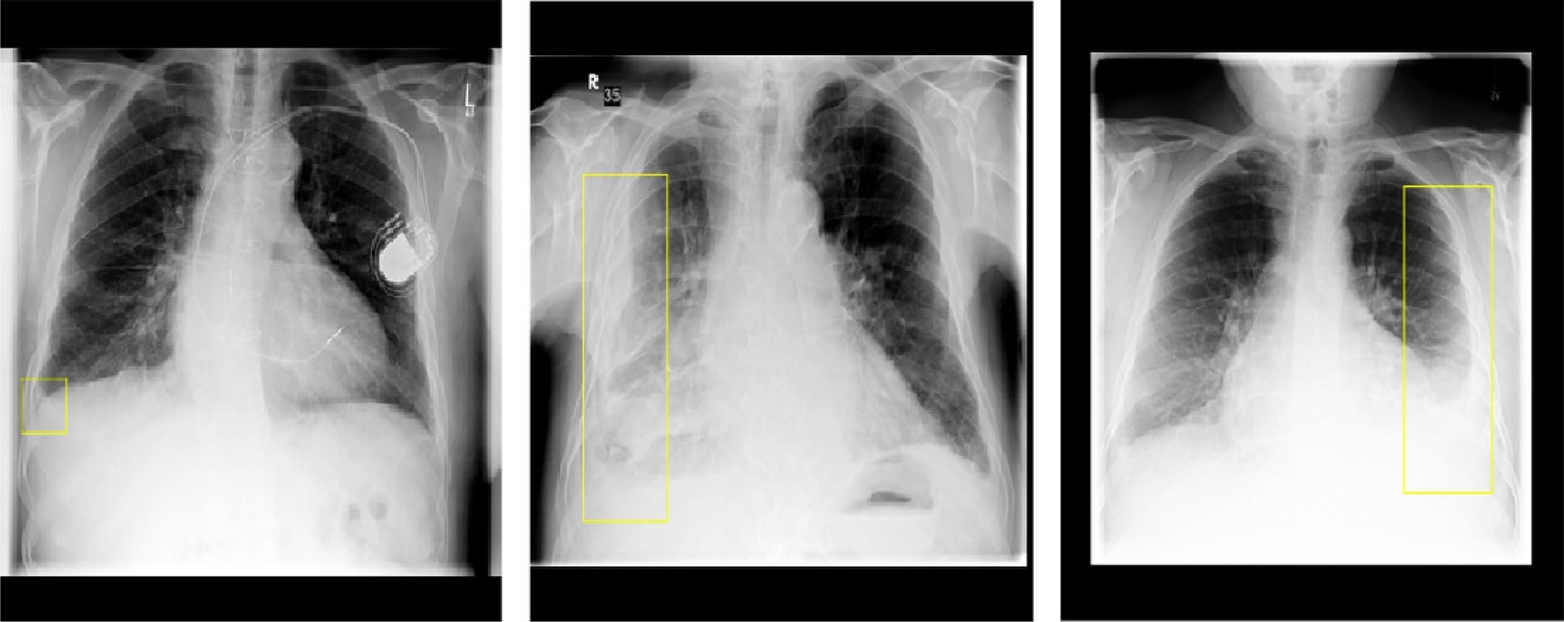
Example Chest X-Rays, sourced from the internal dataset CXR_16k, display pleural effusion abnormalities identified by bounding boxes.
